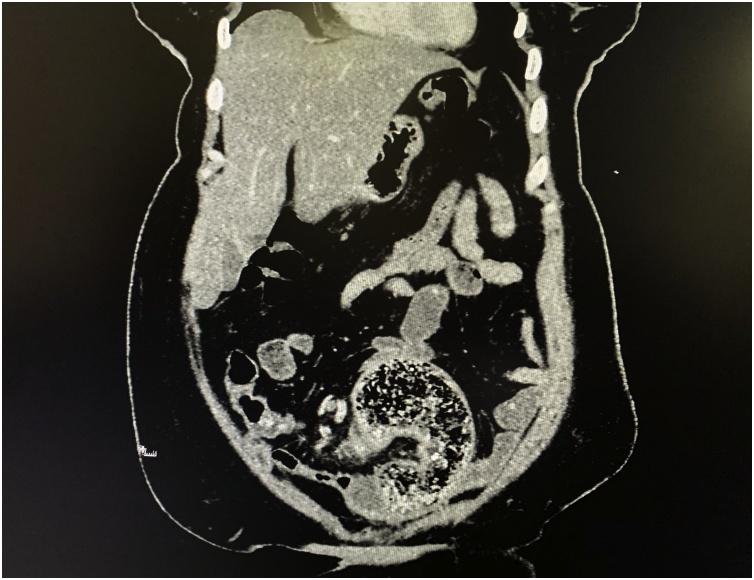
CT-scan of the abdomen showing an evidence of thickened wall of the sigmoid colon with intramural air, the proximal small bowel show dilatation.
